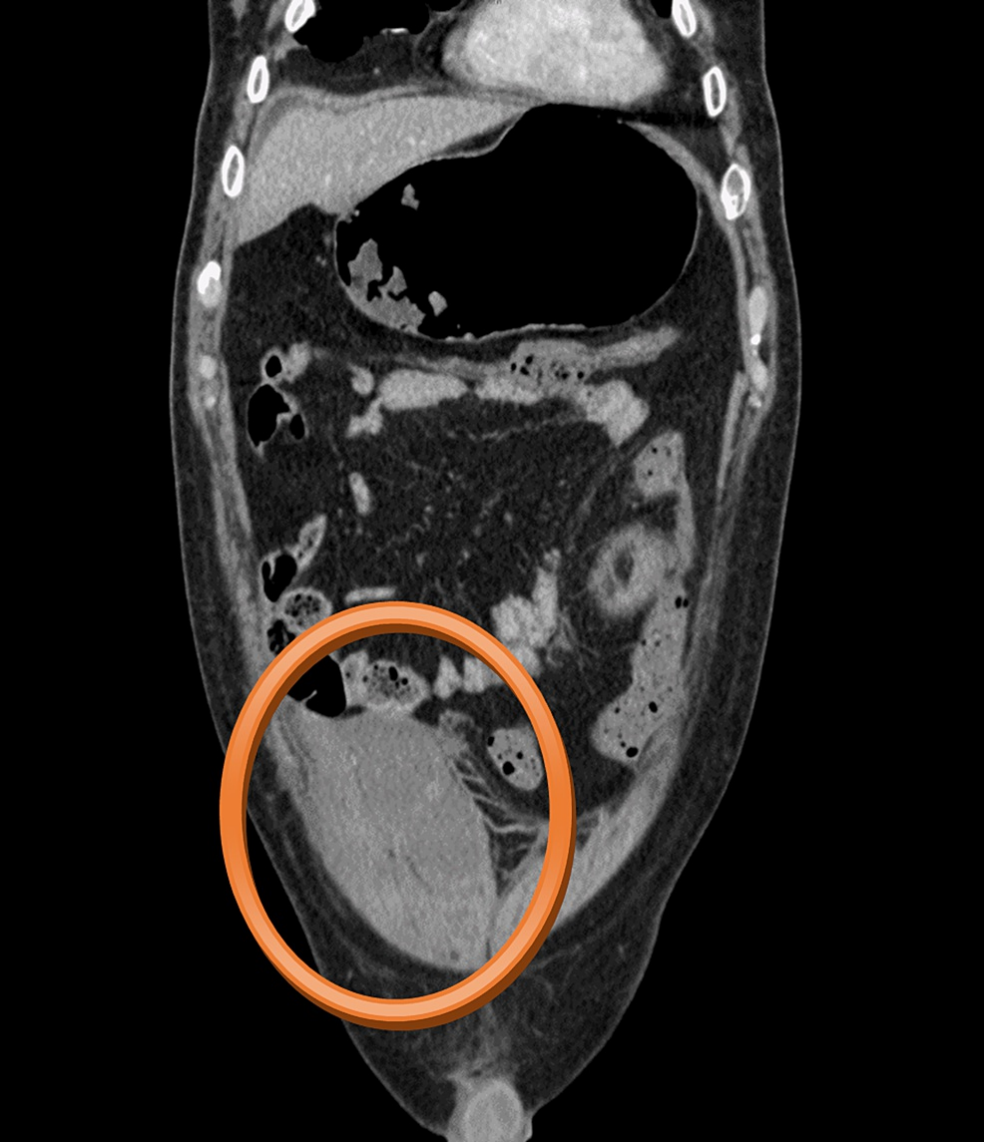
CT scan - coronal plane Hematoma of size 130 x 80 x 50 mm in the right musculus rectus abdominis can be seen
